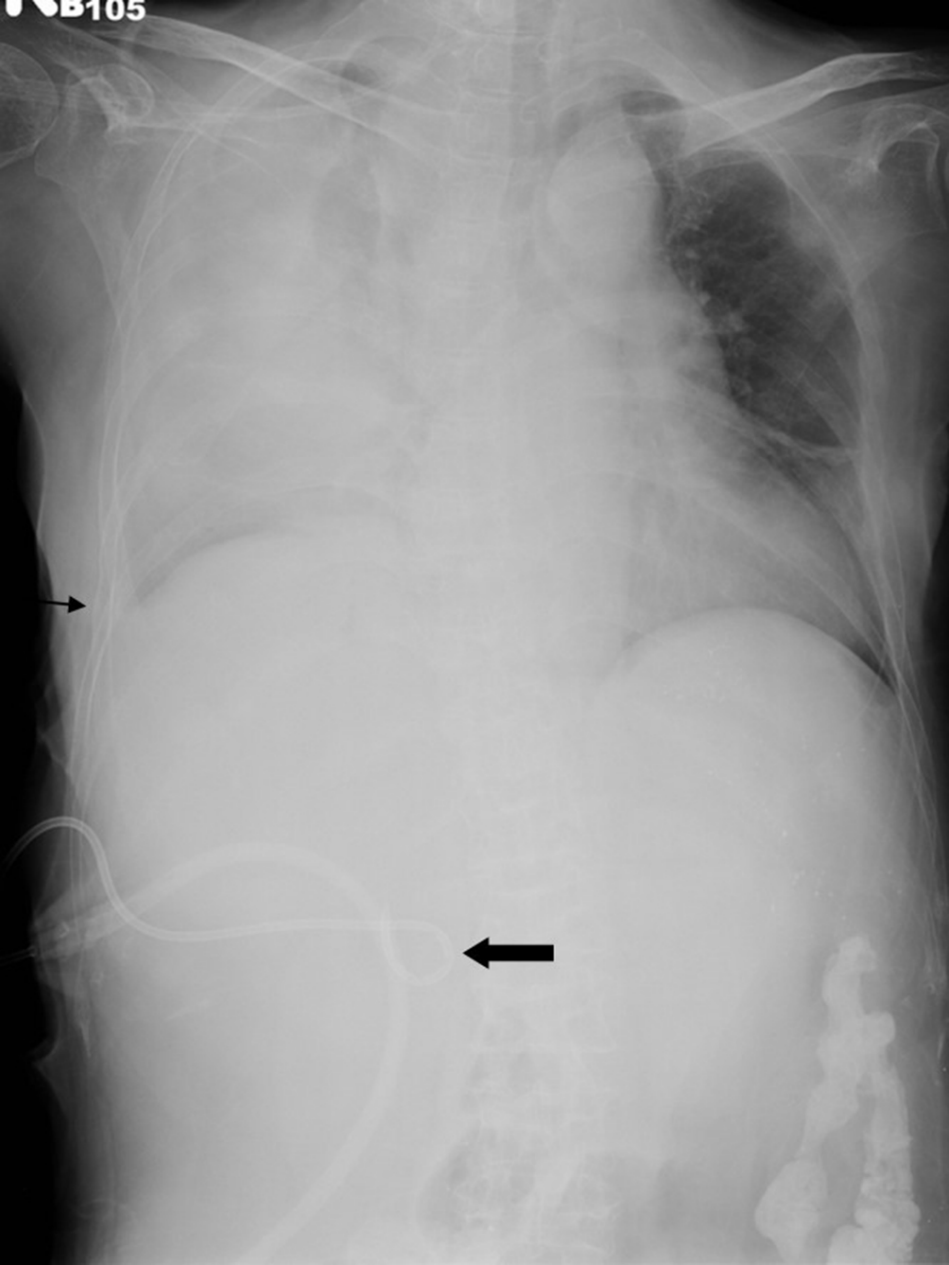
Chest X-ray film reveals opacification of the right lung, blunting of the right costophrenic angle (thin arrow) and partial migration of the catheter (thick arrow).
